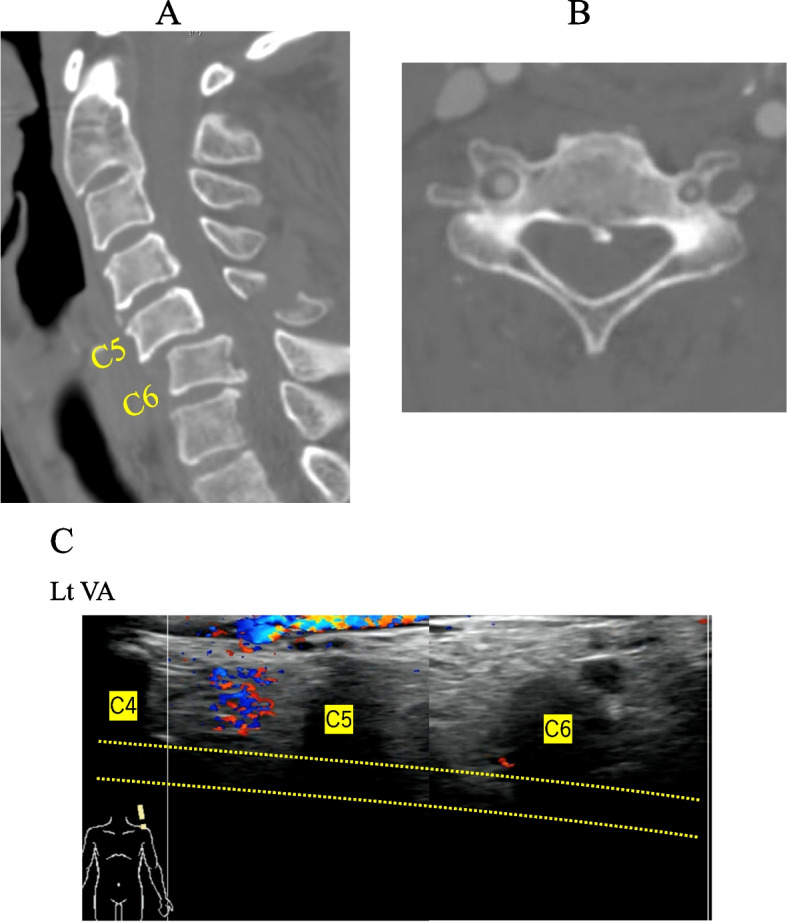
A Computed tomography shows the dislocation fracture at C5/C6. B CTA shows the stenosis of the left VA at C5 level. The diameter of VA on the left side was 45.9% compared with the one on the right side. C Flow of the VA in the left side not seen on US. Part of VA that originally passed through is indicated by dotted lines
